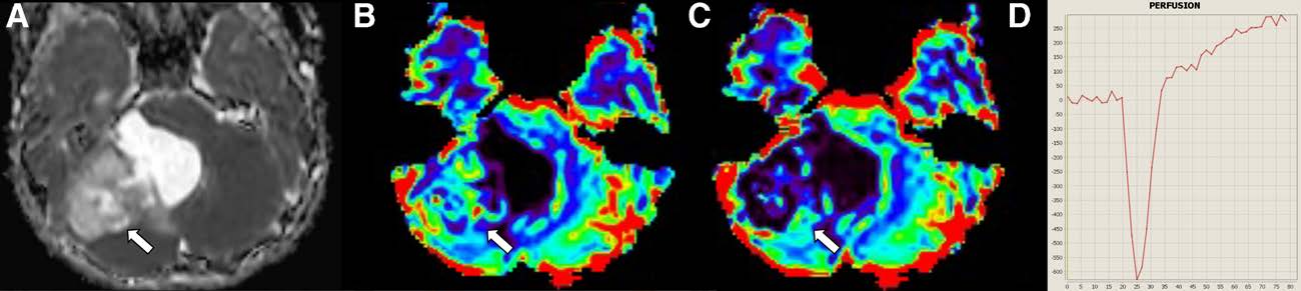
Pilocytic astrocytoma in an 11-year-old boy. MRI shows a solid cystic mass in the right cerebellar hemisphere. Normalized mean apparent diffusion coefficient is 1.94 (a, arrow). Normalized relative cerebral blood flow and volume are 2.07 and 2.28, respectively (b and c, arrows). The percentage signal recovery is 141.6 (d). MRI = magnetic resonance imaging.
